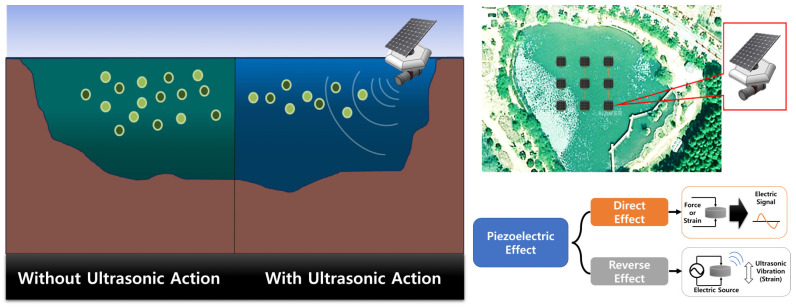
Ultrasonic action in the proposed algae removal system.
